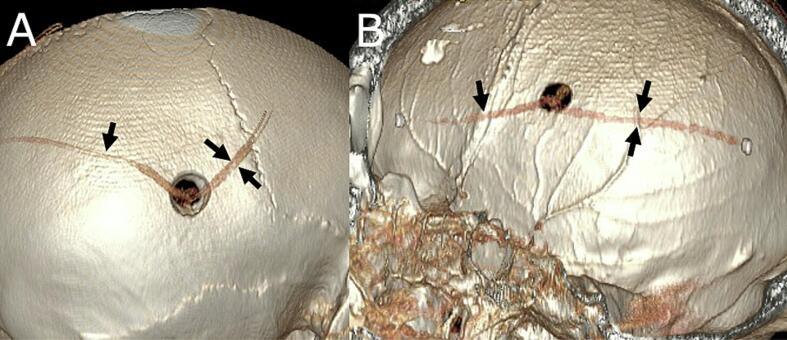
A, B: Reconstructed postoperative cranial CT images. Two drains are inserted anteriorly and posteriorly into the subdural space from a burr hole in the left parietal region. One drain (indicated by the arrow) is used for medication infusion, while the other (indicated by the paired arrow) discharges effluent.
